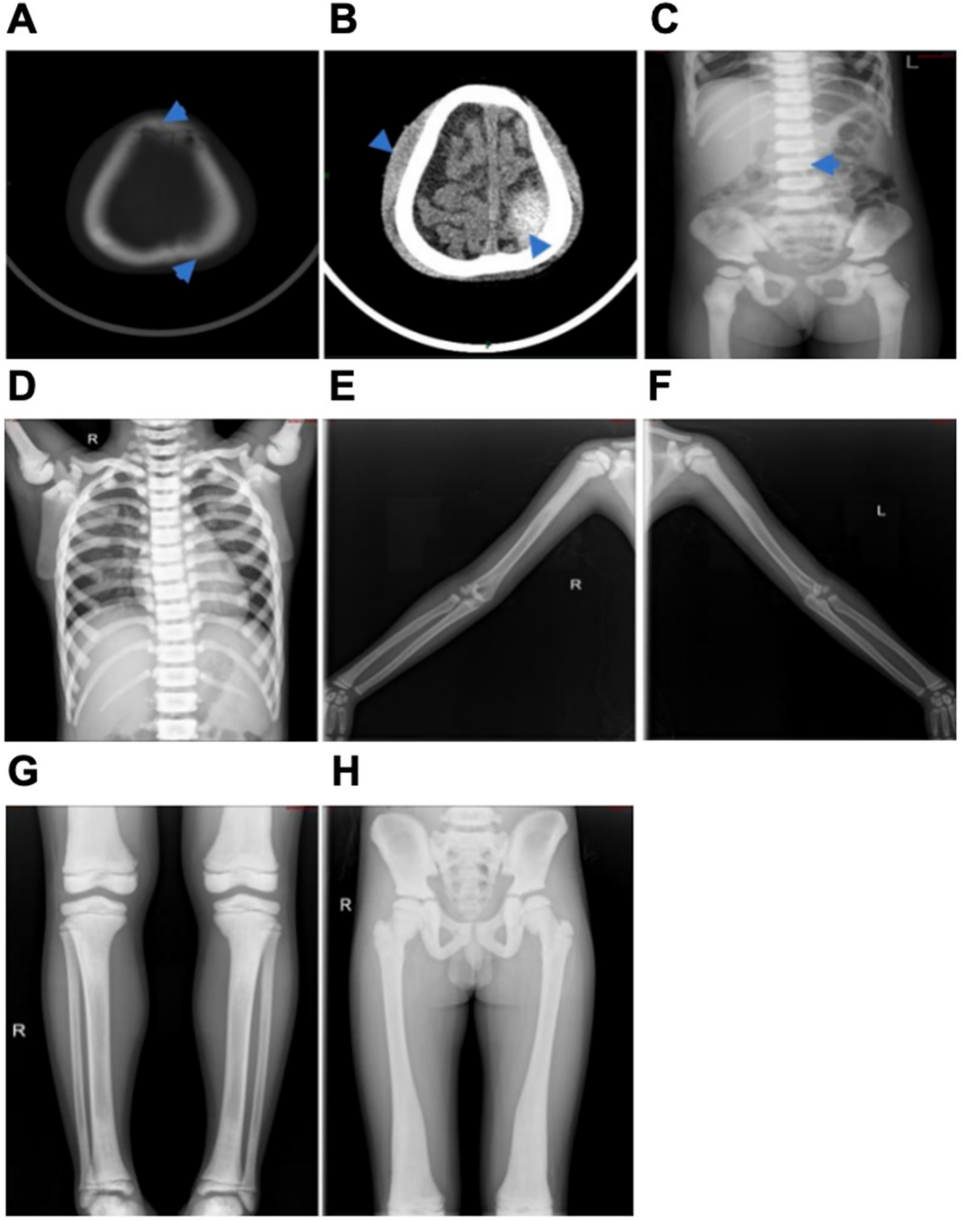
Abdominal x-ray film of the proband of patient 1 (A–C) and x-rays of the proband of patient 2 (D-H). (A) The top arrow represents a frontal fracture and the bottom arrow represents a left parietal fracture. (B) The top arrow represents a scalp hematoma and the bottom arrow represents epidural blood. (C) Arrows represent diffuse increased density of the spine, ribs, pelvis, and proximal femur, marked hardening of the upper and lower vertebrae, flattening of the vertebrae, and “sandwich-like” changes. (D) The diffuse density of the bones in the film increased, and the vertebral body was “sandwich-like” change. (E,F) The bone density of both humerus increased, the bone cortex thickened, and the medullary cavity narrowed. (G) The distal femur and proximal tibia showed “flakelike” changes. (H) The bone density of pelvic bones increased significantly, the bone cortex thickened, the bilateral femoral bone marrow cavity was not clear, and the iliac bone showed “bone in bone”.
